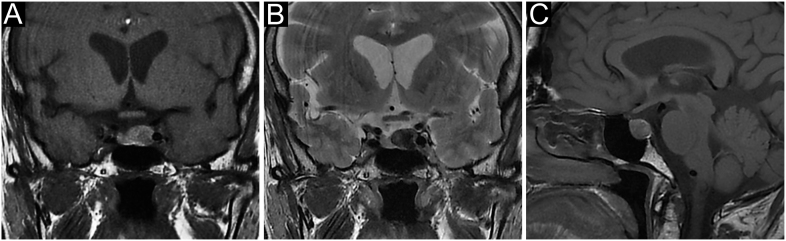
An intrasellar somatotroph PitNET in a 68-year-old woman. This patient presented with a 3-year history of increasing headache and coarse features, acral enlargement, prognathism and paraesthesia. Elevated plasma GH (5.9 ng/mL) and IGF1 (510 ng/mL, 1.8 × ULN) confirmed acromegaly with normal PRL levels (7.7 ng/mL). A 15 mm intrasellar, non-invasive pituitary tumour was found at MRI, hypointense on T2-weighted scans. She had a long-standing thyroid goitre, hypertension and impaired fasting glucose, a recent diagnosis of carpal tunnel syndrome and a recent unexplored breast mass, which was immediately referred for biopsy, revealing a ductal carcinoma. Thus, she received first-line somatostatin receptor ligands treatment to allow surgery and radiotherapy for breast cancer, and GH/IGF1 was already controlled when she underwent transsphenoidal surgery. Pathological examination concluded for a GH/PRL-secreting PitNET. After post-operative remission, cabergoline was recently introduced (1.5 mg/week) for slightly elevated IGF1 (1.2 ULN) and anti-aromatase treatment is ongoing with no evidence of oncological recurrence. (A) Coronal contrast-enhanced T1-weighted scan, (B) coronal T2-weighted scan and (C) sagittal contrast-enhanced T1-weighted scan.
